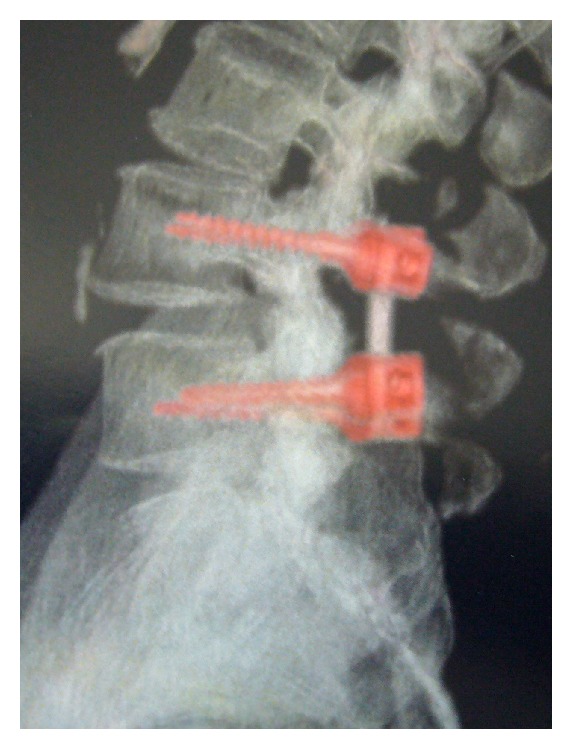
CT scan of another 71-yo patient with bone window shows clearly the PEEK rod and can detect a possible rupture.
